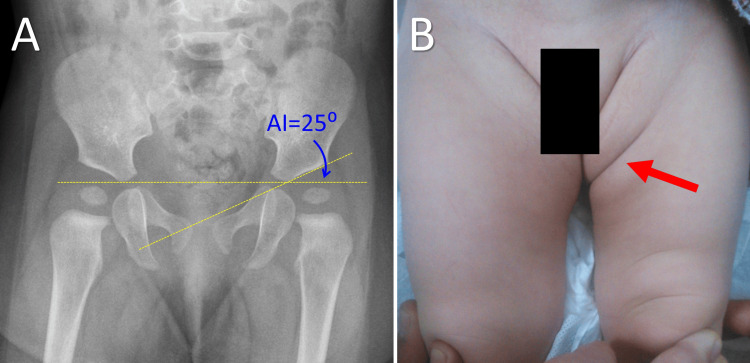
Asymmetric skin fold (ASF) formation in a five-month-old infant with healthy hips A. An anteroposterior X-ray projection of the pelvis. No signs of dysplasia of the left hip are evident. The acetabular (AI) index is 25 degrees; B. A huge, deep ASF is manifested on the left thigh (red arrow). Image credits: P. Samelis
